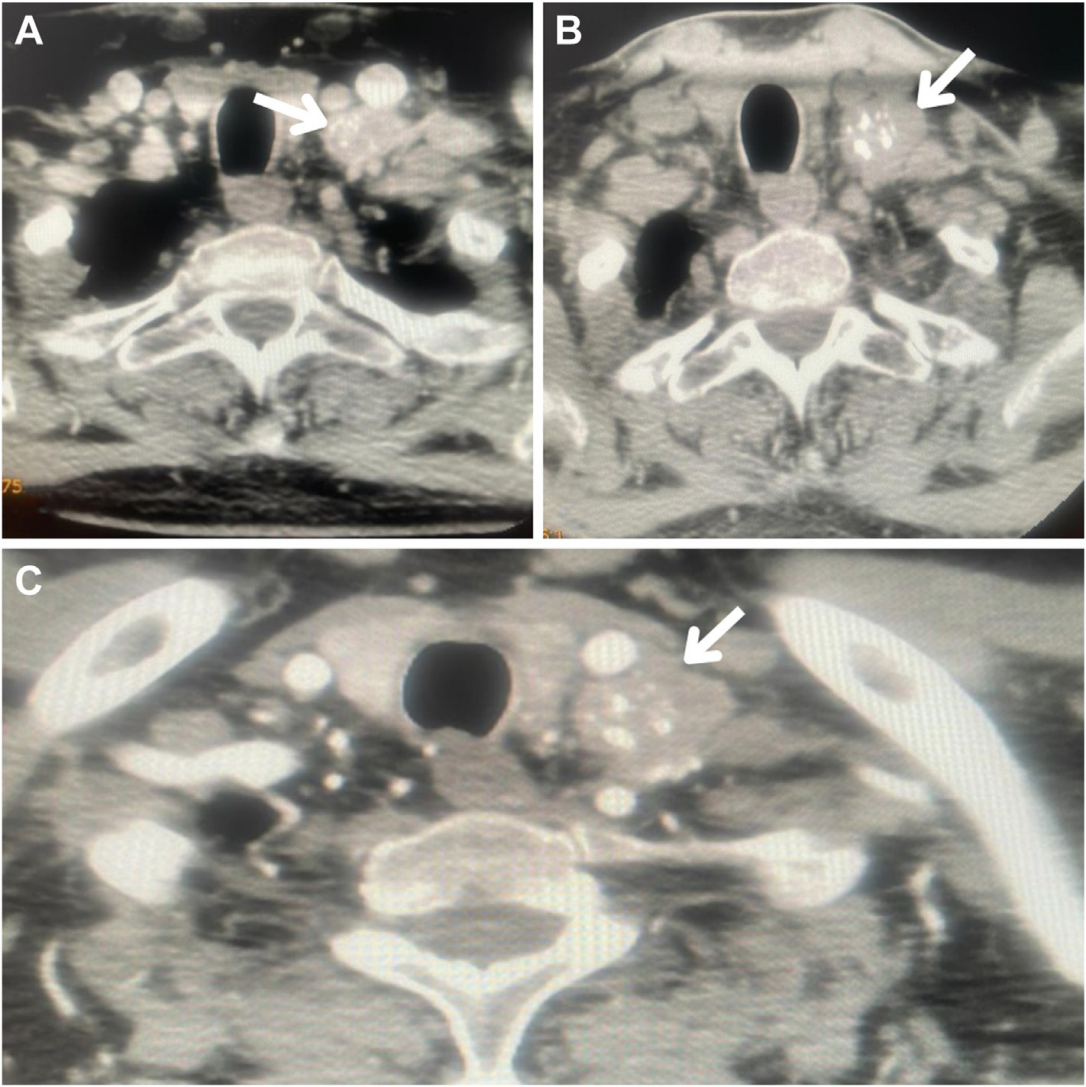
Neck computed tomography showing multiple enlarged lymph nodes seen in the central and left lateral compartments. No pathologic lymph nodes were identified on the right side. White arrows indicate the suspicious lymph nodes in (*A*-*C*).
